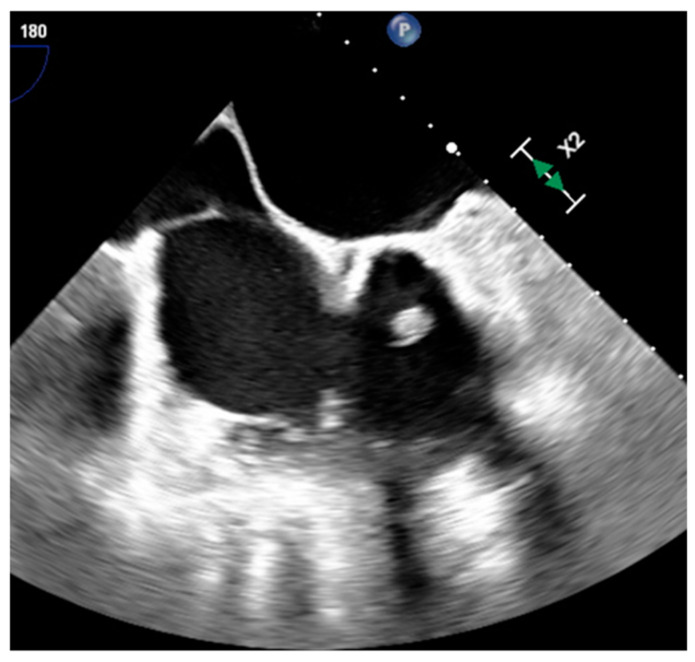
A prominent eustachian valve at transesophageal echocardiography.
